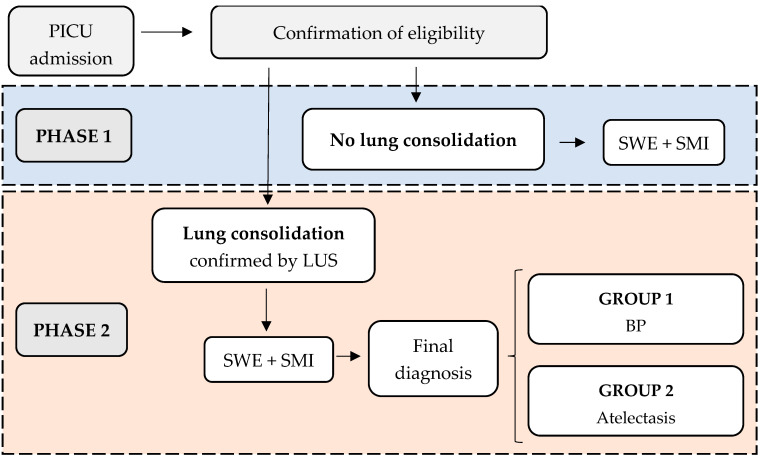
ELASMIC study phases. PICU: Paediatric Intensive Care Unit; LUS: Lung Ultrasound; SWE: Shear Wave Elastography; SMI: Superb Microvascular Imaging; BP: Bacterial Pneumonia.
